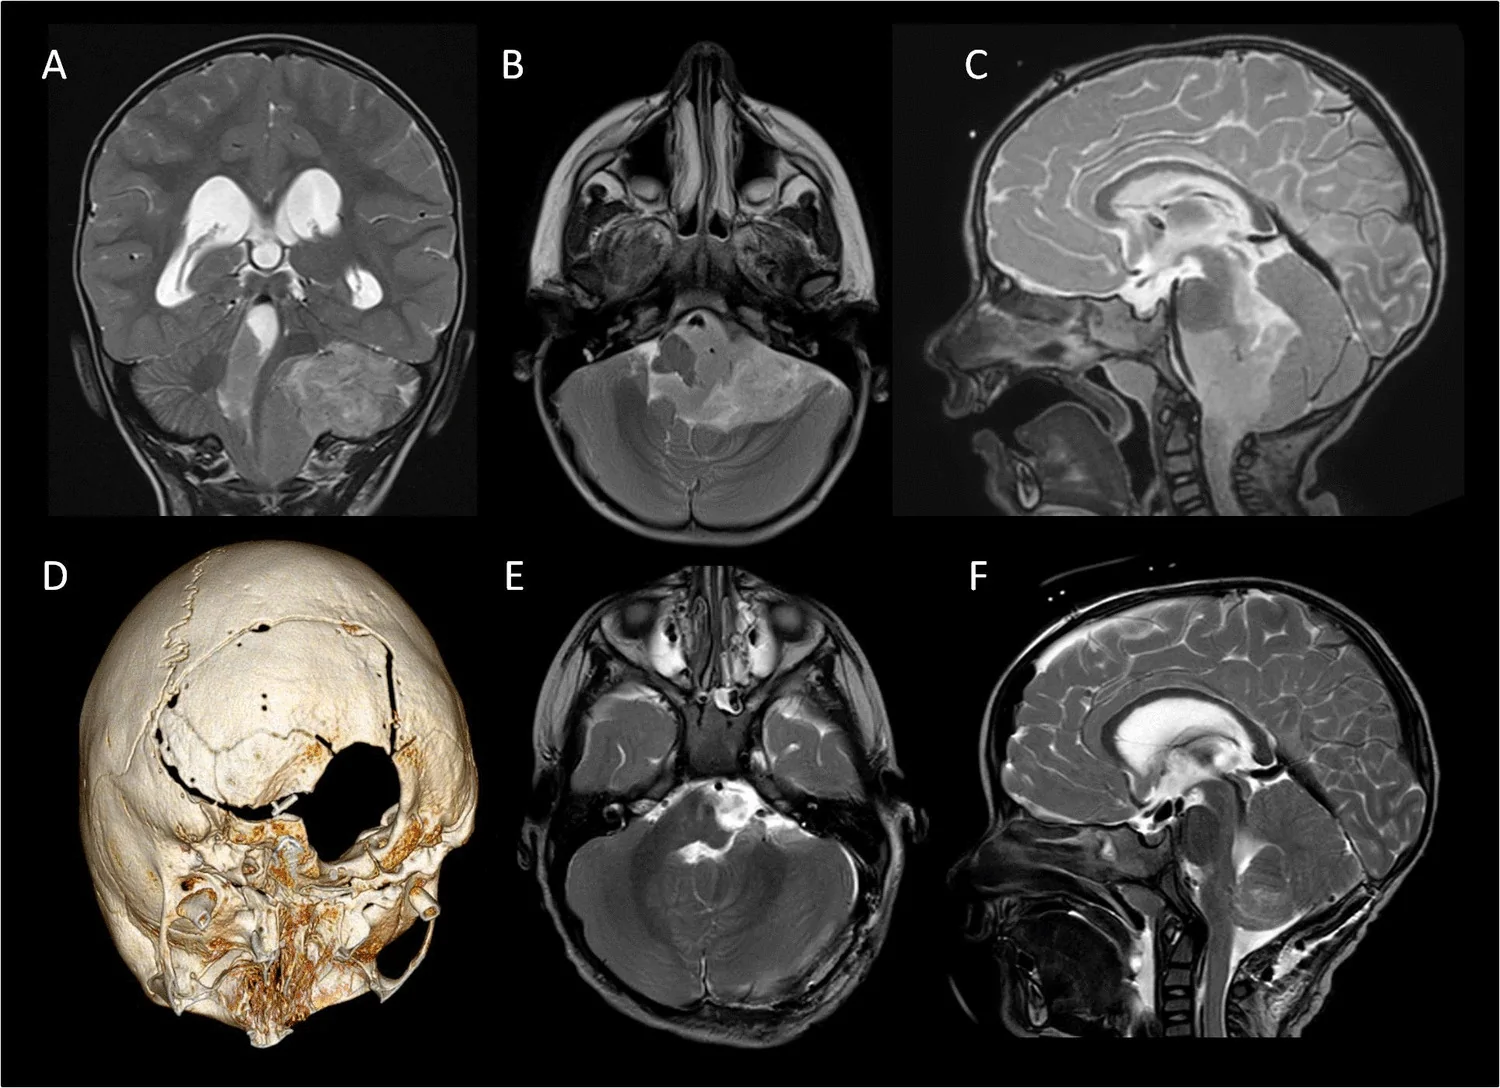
Preoperative coronal (A), axial (B) and sagittal (C) T2- weighted MRI images. Note the tumor extending from the foramen of Luschka into the fourth ventricle, cerebellopontine angle and in front of the brainstem. D — computer tomography reconstruction shows the extent of the craniotomy. Corresponding postoperative MRI in axial (E) and sagittal (F) planes confirming gross total resection
